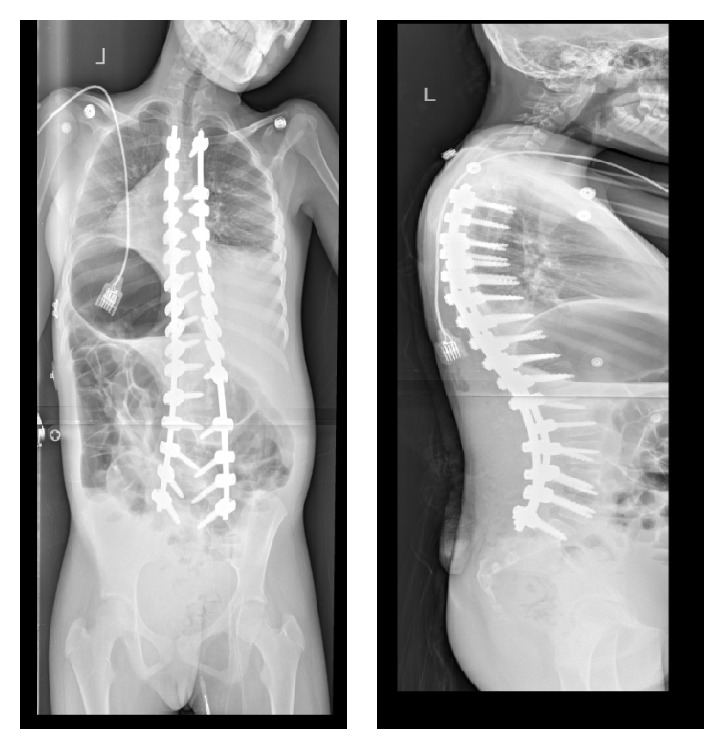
Immediate postoperative radiographic images showing excellent correction of the deformity. Patient is well-balanced in coronal and sagittal planes. The pelvis and shoulder are level.
